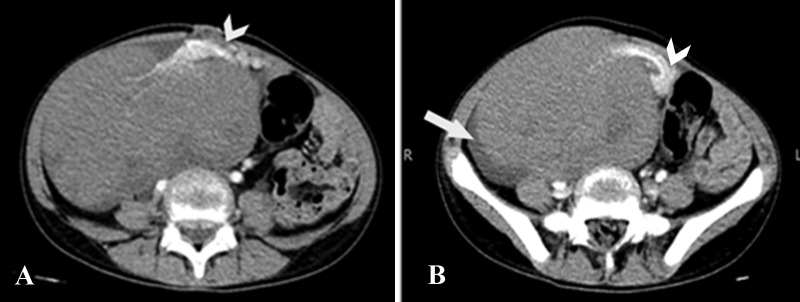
Computed tomography of the abdomen and pelvis axial sections A & B: Twisted vascular pedicle in the medial aspect of the mass, which was originating from the left adnexa (arrowheads). Free fluid seen adjacent to the lesion (arrow).
